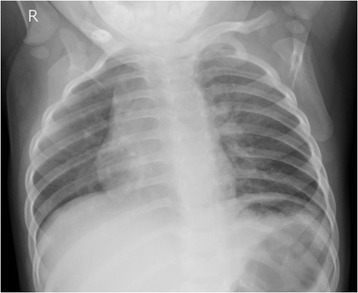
Chest X-ray images taken prior to the surgery
